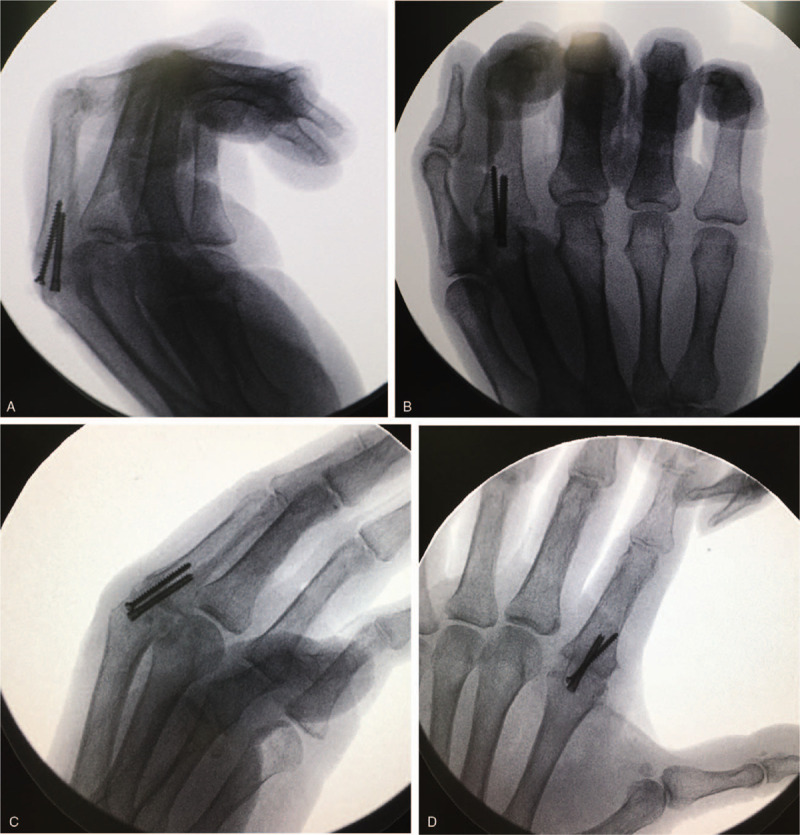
MCP joints were immobilized with screws in 30° of flexion. (A and B) Lateral and anteroposterior X-rays of the right hand. (C and D) Lateral and anteroposterior X-rays of the left hand.
